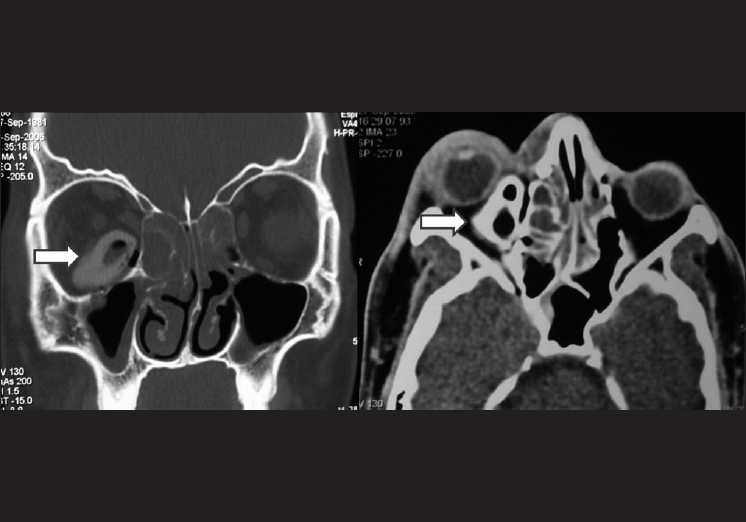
Computed tomography scan showing the intraorbital plastic foreign body in the right orbit with the air pockets (arrows)
